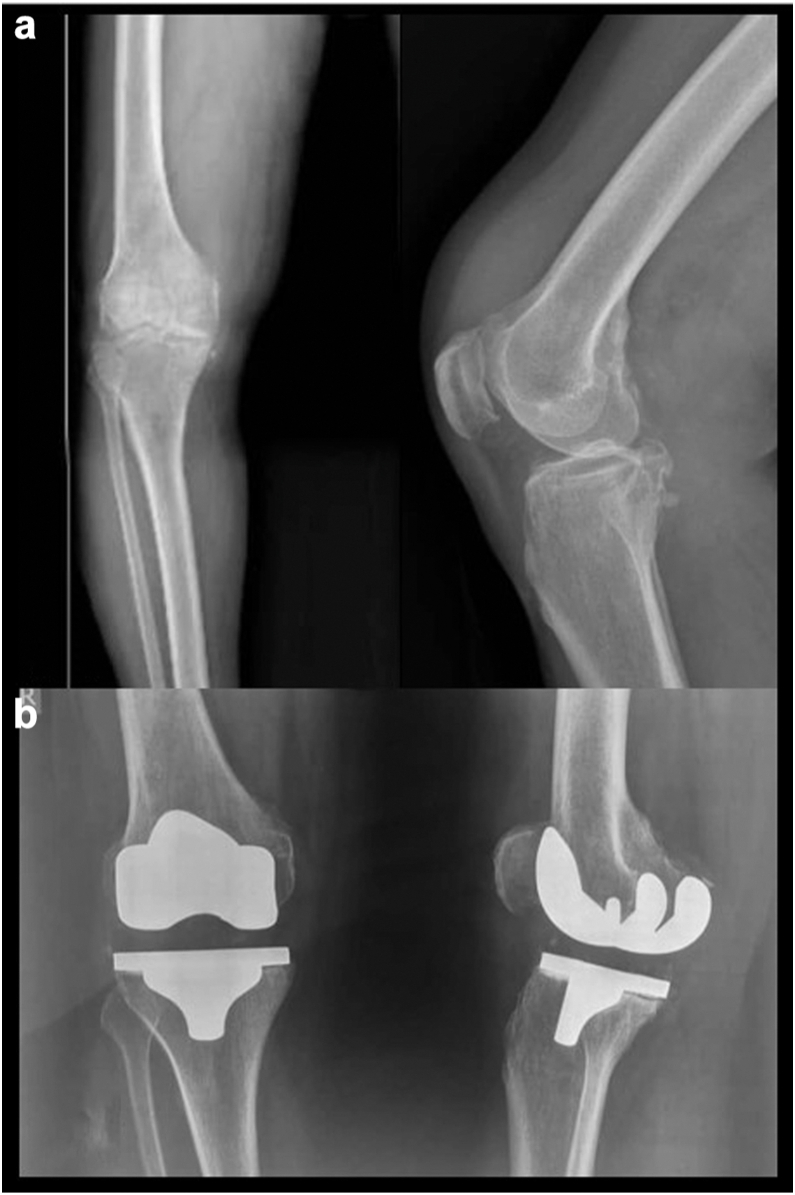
(a) Preoperative anteroposterior and lateral radiographs of the right knee in case 2, showing end-stage osteoarthritis with marked joint space narrowing, marginal osteophytes, and subchondral bone changes. (b) Postoperative anteroposterior radiograph at 24 months demonstrating stable unilateral total knee arthroplasty with well-aligned and securely fixed components.
